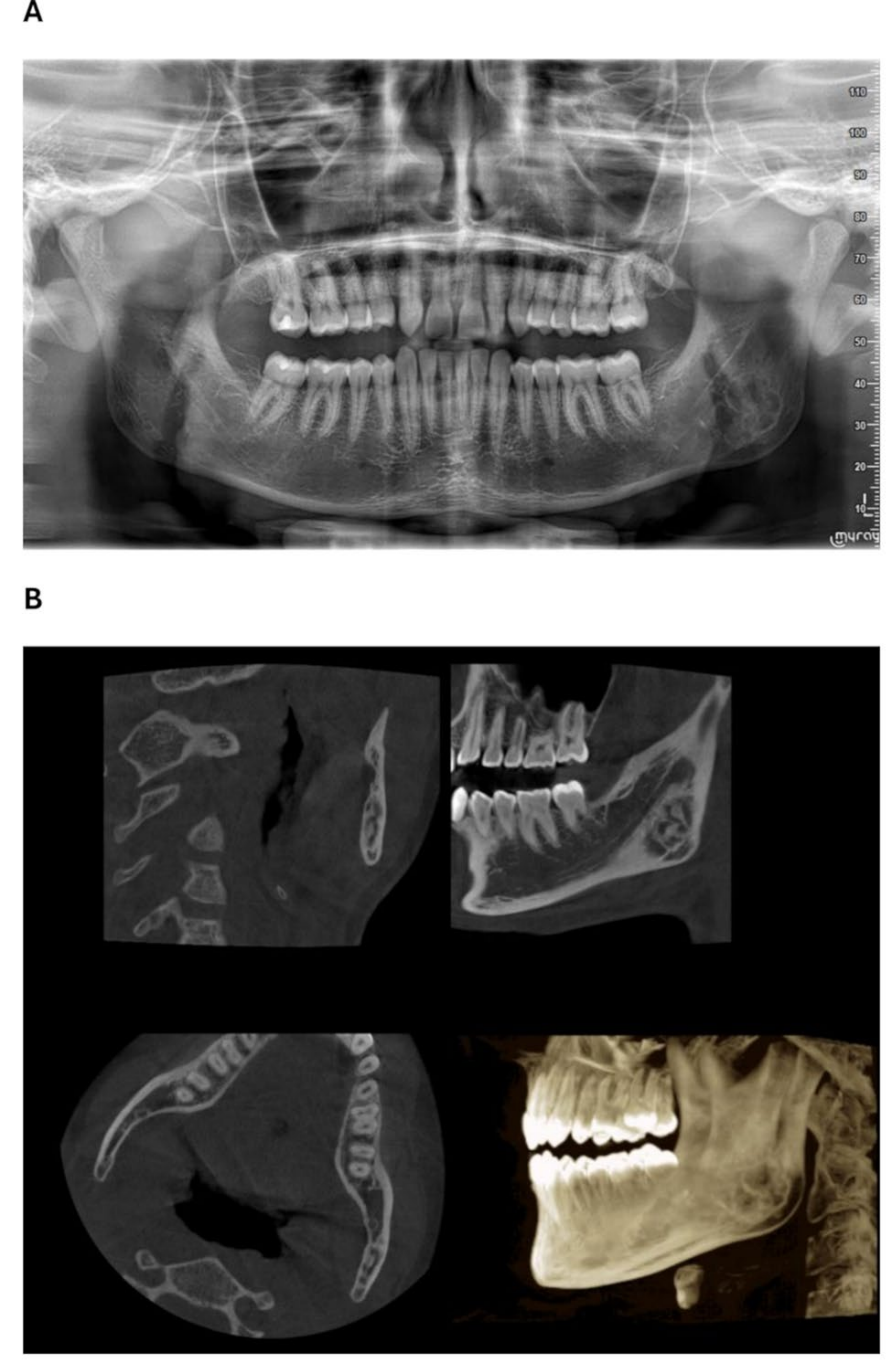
A: Initial panoramic radiograph showing an osteolytic lesion of the left mandibular angle. B: Initial cone beam computed tomography images: multipartitioned osteolytic lesion of the left mandibular angle
